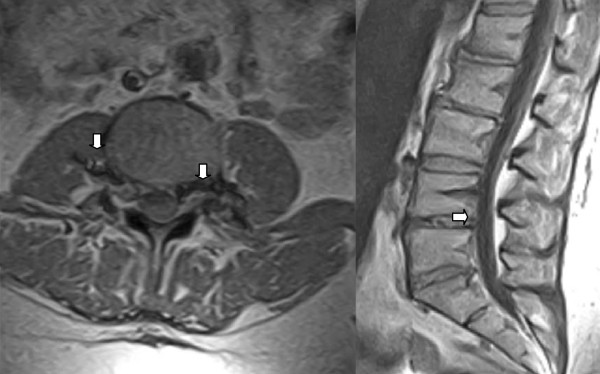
Epidural varicosis (arrows). MRI scan of the lumbar portion (transversal and sagittal).
